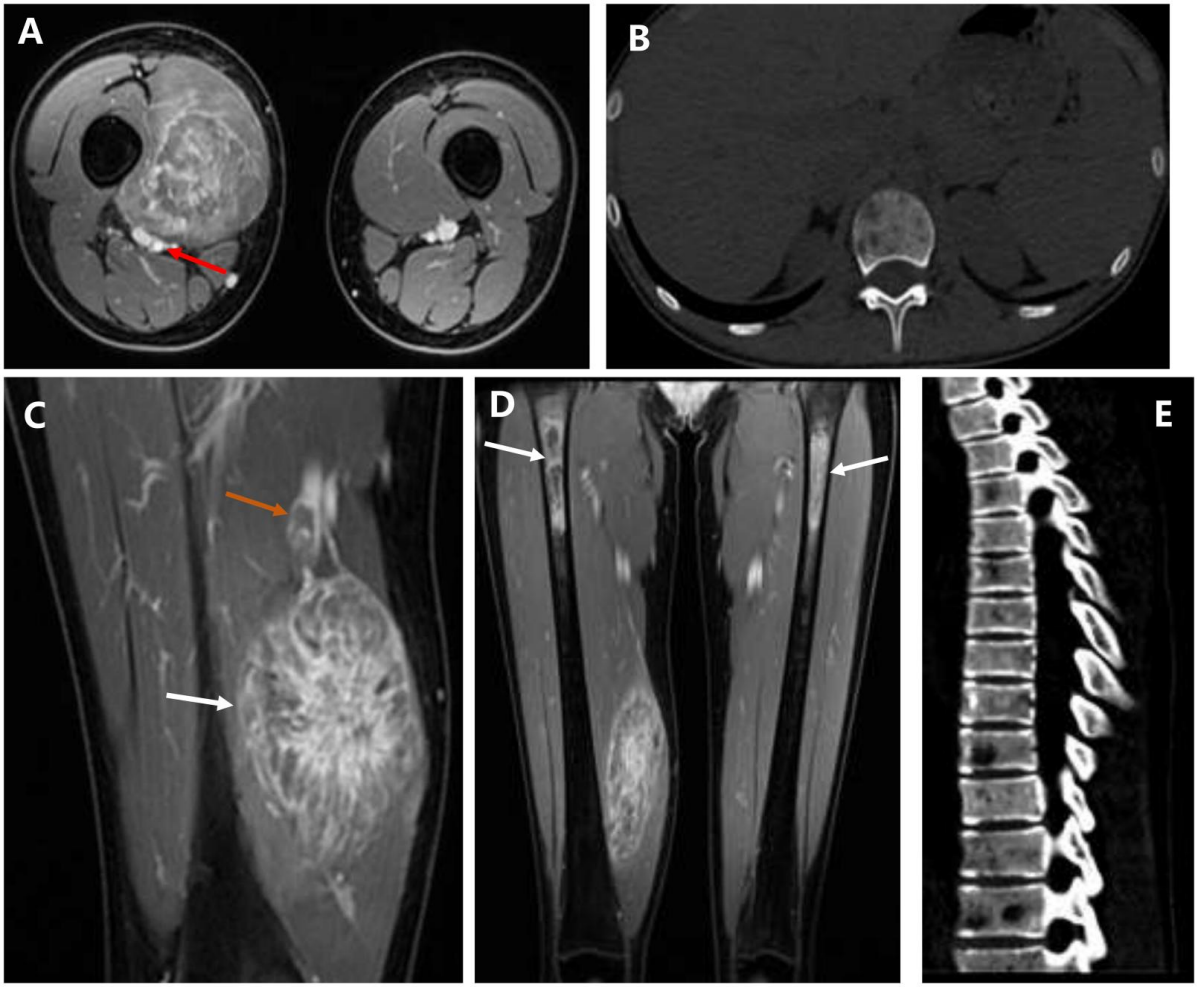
Contrast-enhanced MRI (A, C, D) and CT (B, E) of the ARMS. (A, C, D) Contrast-enhanced MR imaging showed marked heterogeneous enhancement of the mass in the right vastus medialis muscle, which manifested as a distinctive “chrysanthemum petal-like” morphology created by radiating enhancing septa. (A)The femoral artery posterior to the mass was displaced and closely abutted by the lesion (red arrow). (C) Sagittal MRI shows pseudocapsule enhancement at the edge of the mass (white arrow). An intraluminal filling defect demonstrating heterogeneous enhancement was identified within the femoral vein (orange arrow). (D) Coronal MRI showed multiple patchy abnormal enhancements in the bilateral proximal femoral diaphyses (white arrows). Plain CT scan of chest (B, bone window) and Sagittal reformatted CT scan images of the thoracolumbar spine (E, bone window) showed multiple lesions of low-density bone destruction in the thoracolumbar spine.
